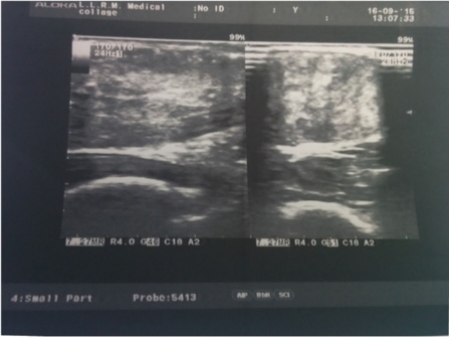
Ultrasonography of tendo-Achilles showing anteroposterior thickness of 7.5 mm with loss of normal tendon appearance and multiple hypoechoic foci within tendon
